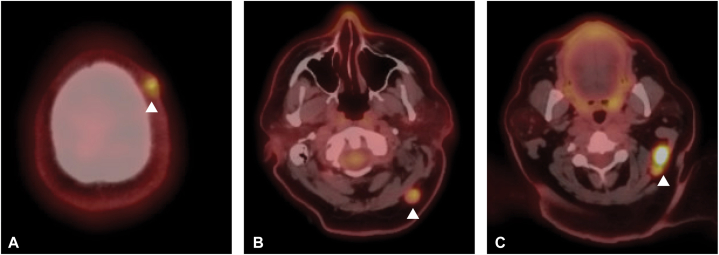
PET/CT imaging of the head and neck shows FDG-avid lymphadenopathy of the postauricular and occipital nodes, and an incidental FDG-avid lesion (arrowheads). (A) Incidental identification of a secondary scalp lesion, suspected to be unrelated to a proliferating trichilemmal tumor. (B) FDG-avid occipital node positive for adnexal carcinoma on lymph node dissection. (C) FDG-avid postauricular node positive for adnexal carcinoma on lymph node dissection.
